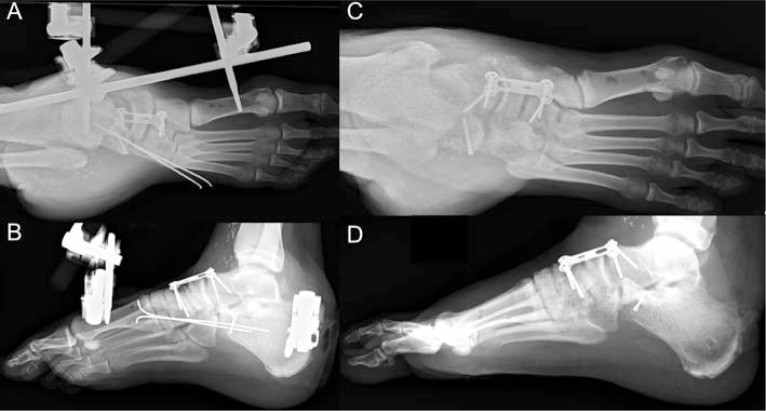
Radiographs at 6 weeks (A, B) and 3 months (C, D) post-operatively showing cell-reduced calcaneocuboid and naviculocuneiform joints. The external fixator was removed after 6 weeks and the patient was allowed to bear weight at 10 weeks.
